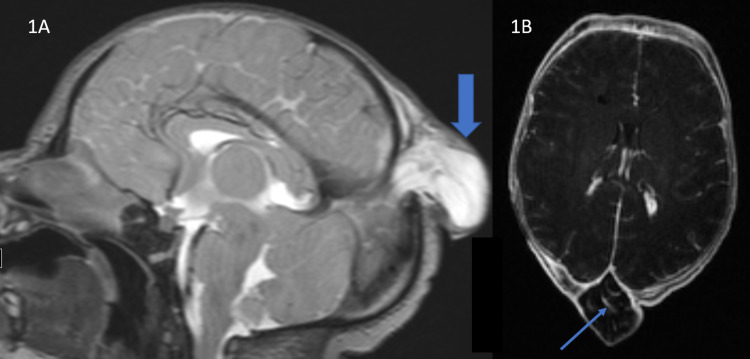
Parietal encephalocele T2-weighted sagittal MRI image (A) of the brain shows a bony defect in the midline with protrusion of dysplastic brain, CSF, and meninges through this defect consistent with parietal encephalocele (thick arrow). Persistent falcine sinus is present. Superior sagittal sinus is interrupted in the midline due to the encephalocele. T1-weighted axial post contrast MRI image (B) of the brain shows few enhancing vessels (thin arrow) in the dyspastic brain tissue within the parietal encephalocele.
